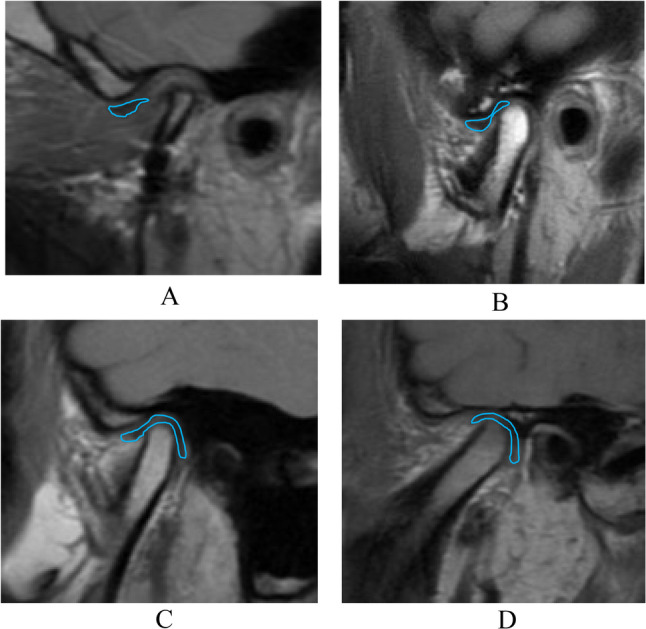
Classification of temporomandibular joint (TMJ) disc position on magnetic resonance imaging. A anterior disc displacement with or without reduction; B anterior type; C fully covered type; D posterior type. Red lines indicate the TMJ disc
